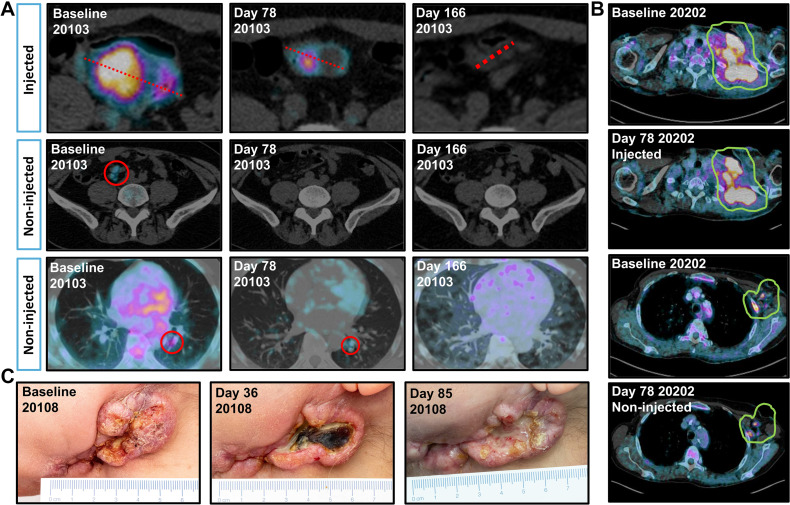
A, Volume and PET signal changes in patient 20103 with metastatic anaplastic thyroid carcinoma, showing disappearance of PET signal for injected abdominal lesion, and disappearance of mesenteric and pulmonal lesions by PET and CT. B, Changes in PET signal in patient 20202 with metastatic NSCLC, showing decrease in PET signal for injected (62% SUVmax decrease) and non-injected lesion (54% SUVmax decrease). C, Visual changes in tumor in patient 20108 with adenocystic adenocarcinoma of the head and neck, showing marked necrosis of the tumor post-treatment.
